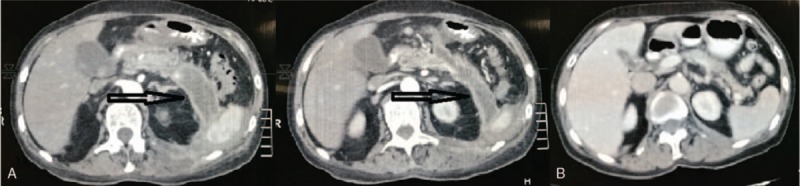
A. Abdominal computed tomography scan depicting retroperitoneal cylinder-shaped fluid collection with thick fibrous wall originating from the pancreatic body and tail and extending to the left flank (arrows). B. Normal follow-up computed tomography scan 2 months after the spontaneous fistula resolution.
